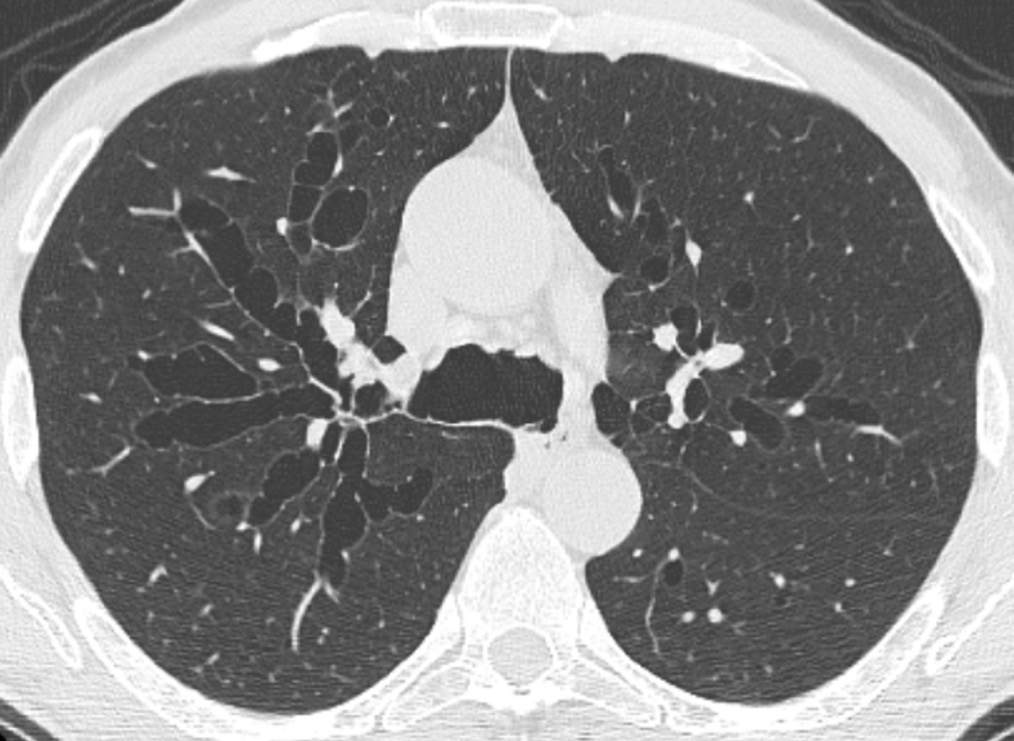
Axial CT image demonstrates enlarged main stem bronchi and thin-walled central bronchiectasis consistent with Mounier-Kuhn syndrome.
